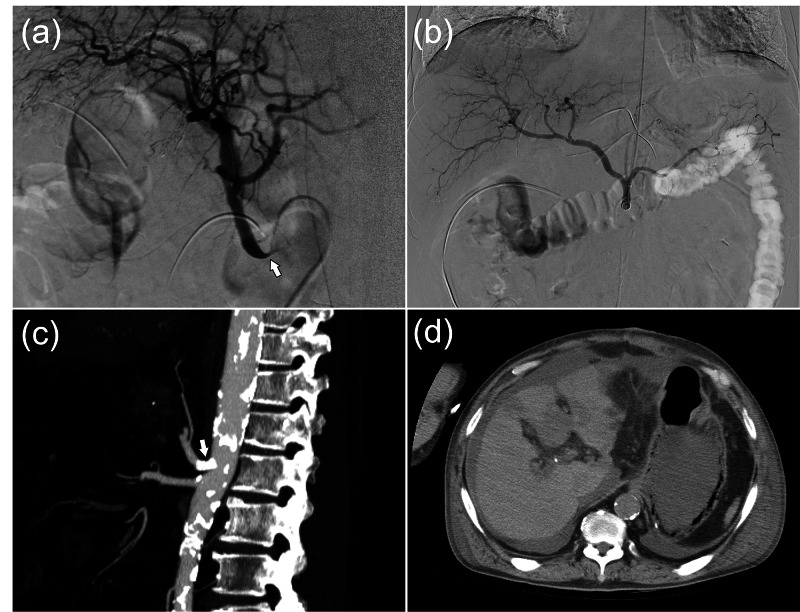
(a) Emergency arteriography showing flexion of the celiac artery (CA) root (the arrow, CA; lateral view). Subsequently, measurement of the CA pressure demonstrated a pressure gradient before and after the stenotic area. (b) Celiac arteriography after bare metal stent insertion. The stenosis was then released. (c) Contrast-enhanced CT on postoperative day (POD) 7 shows CA patency and improved CA blood flow. The arrow indicates the bare metal stent inserted into the CA. (d) Plain CT from POD7 shows gastric emphysema as a change associated with ischemic gastropathy.
